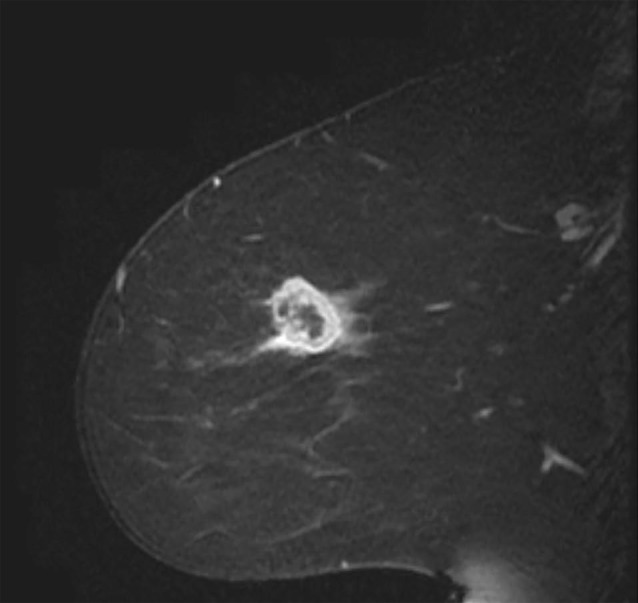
Sagittal T1 post-contrast MRI of a 48-year-old female patient diagnosed with infiltrating ductal carcinoma (ER-, PR-, HER2-) shows an oval rim enhancing mass MRI sequences were obtained from The Cancer Imaging Archive [37].
